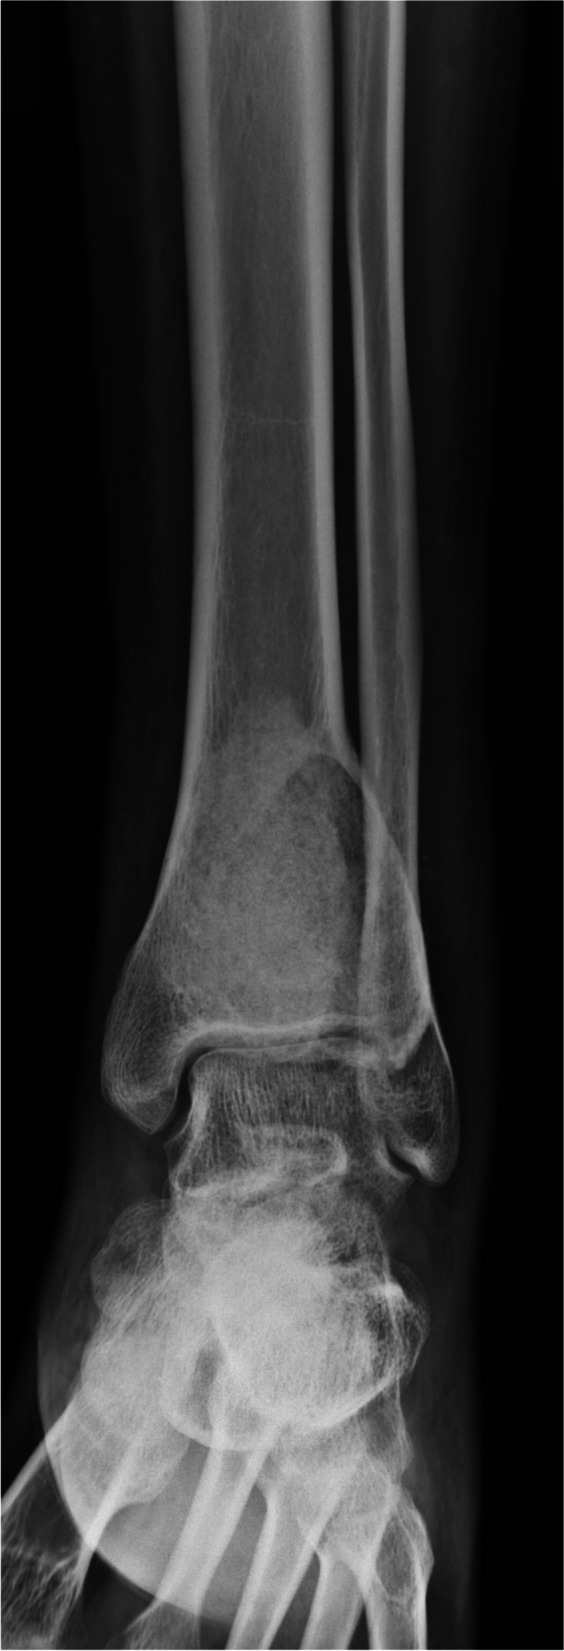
Radiography after filling allograft chip bone following curettage
